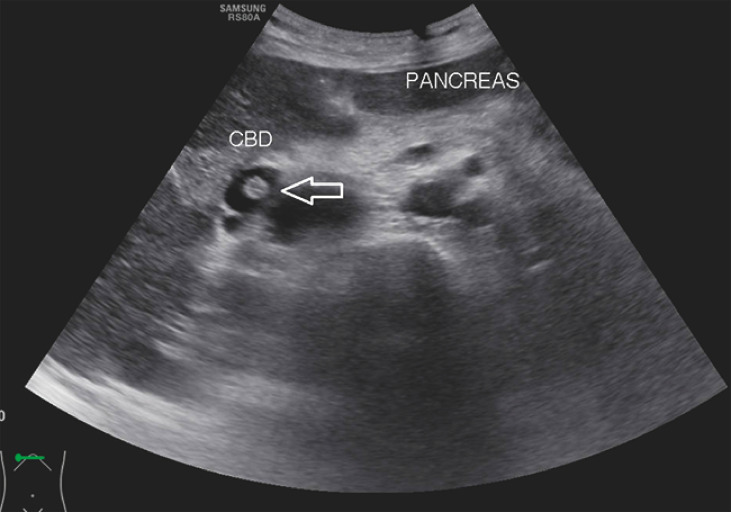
Ultrasonography showed the presence of a worm (arrow) within the biliary tree.
